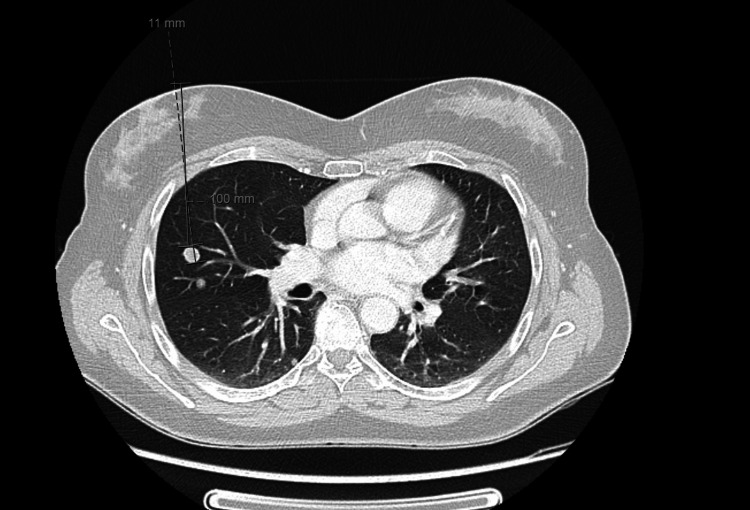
Thoracic CT with multiple lung metastases
